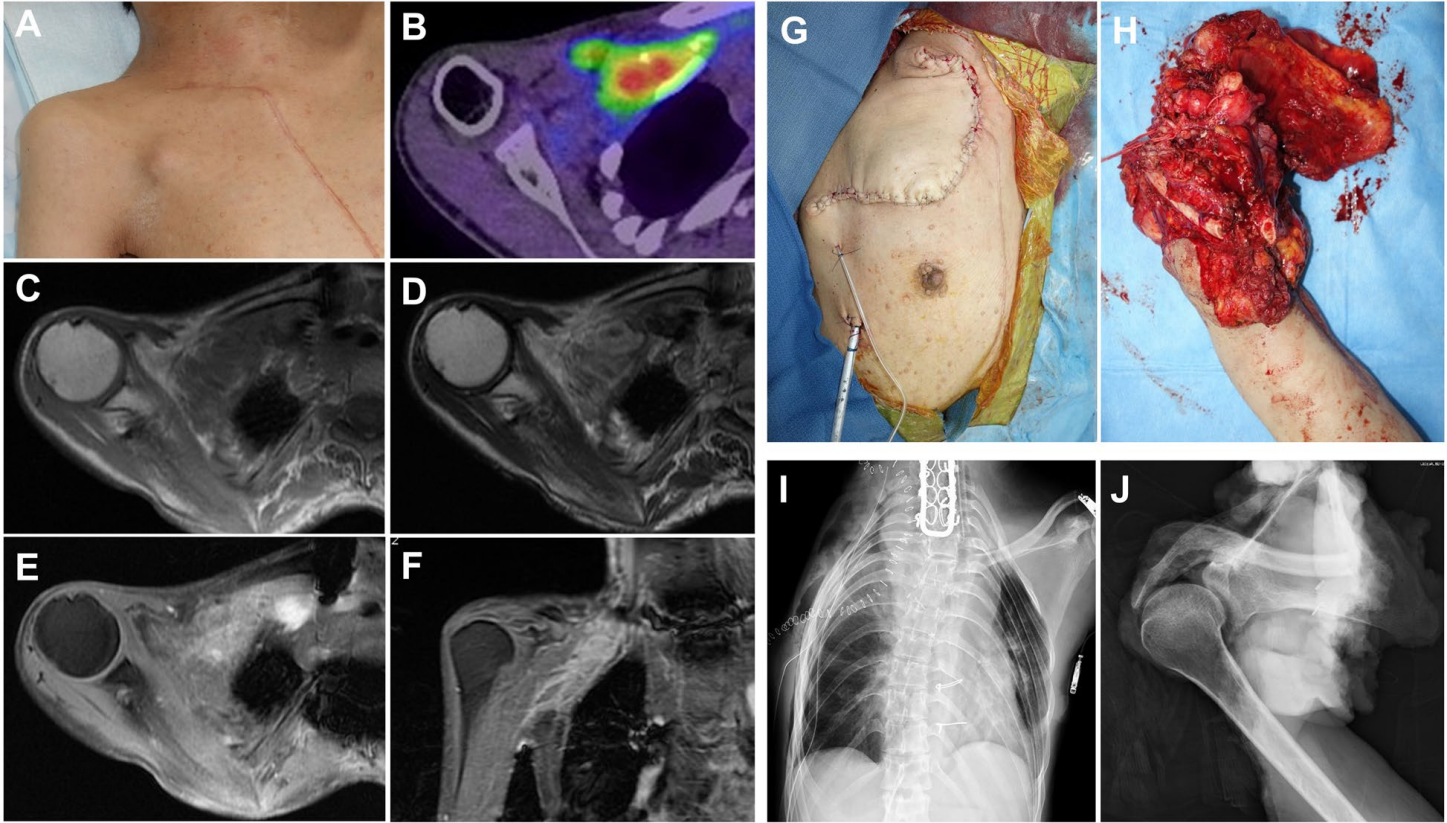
A-F Imaging findings 3 months after thrombectomy. Physical examination shows swelling in the right subclavicular lesion (A), and PET/CT of the chest demonstrates FDG uptake (SUVmax: 7.3) in the tumor (B). MRI shows a significant increase in the size of the remaining recurrent tumor in the subclavicular vein (T1-weighted axial (C), T2-weighted axial (D), fat-suppressed contrast-enhanced T1-weighted axial (E), and coronal (F) sections). G-J Postoperative pictures (G and H) and radiographs (I and J) of the performed forequarter amputation combined with partial chest wall resection
